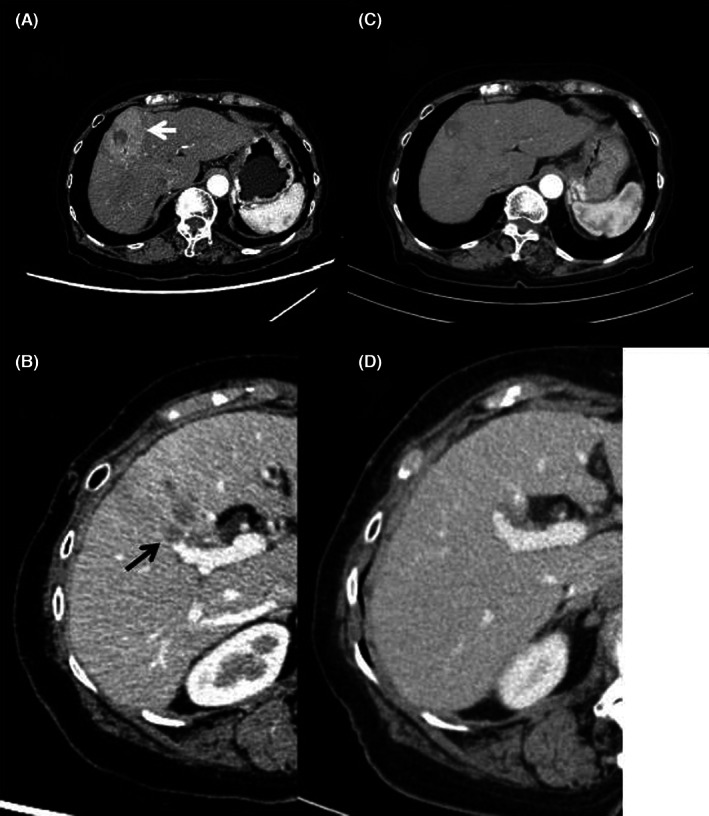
Axial image of contrast‐enhanced computed tomography shows a hypodense tumor with a diameter of about 3 cm in S8 (white arrow) (A) accompanied by portal vein thrombosis in P8 (black allow) (B) before regression in June 2020 and markedly decreased in size with the resolution of the portal vein thrombosis after regression in September 2020 (C, D).
